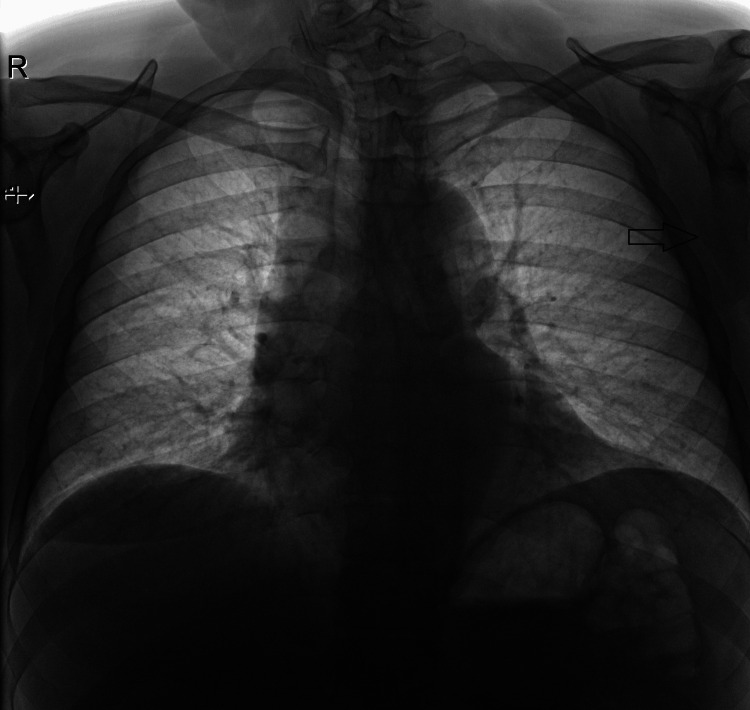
Pre-operative X-ray revealing a shadow in the left axilla
